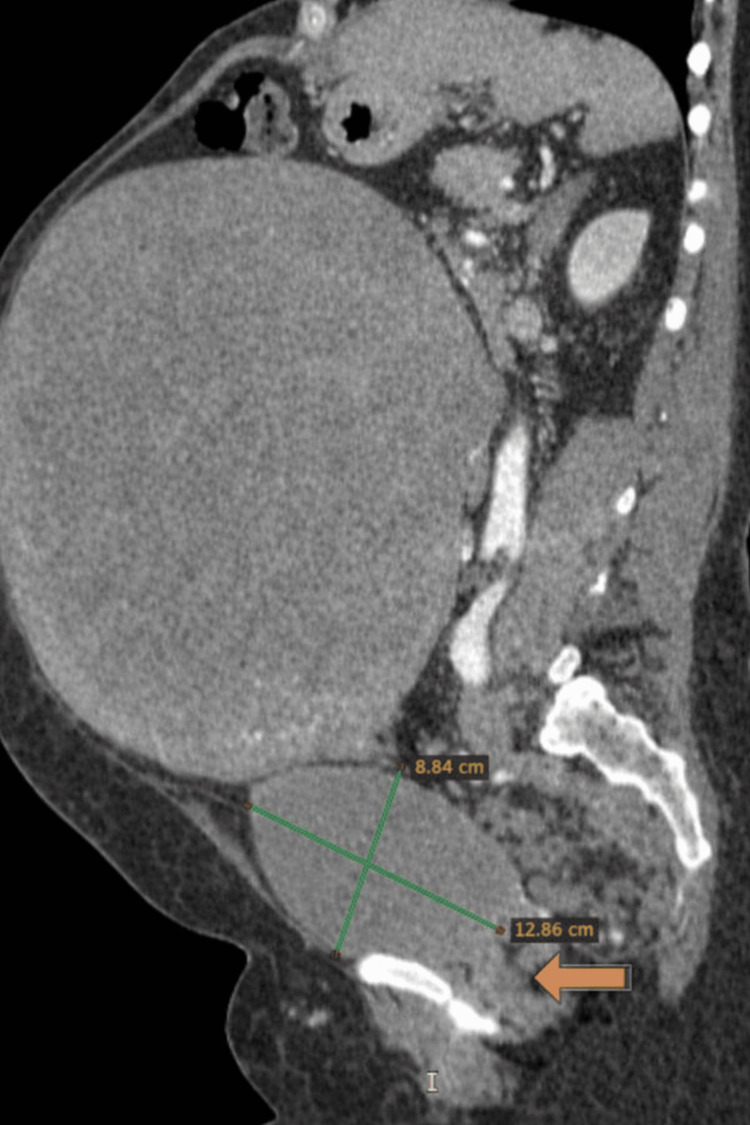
Right parasagittal contrast-enhanced CT image demonstrates caliper measurements of a cystic mass measuring 12.86 × 8.84 cm arising from the right adnexa.
